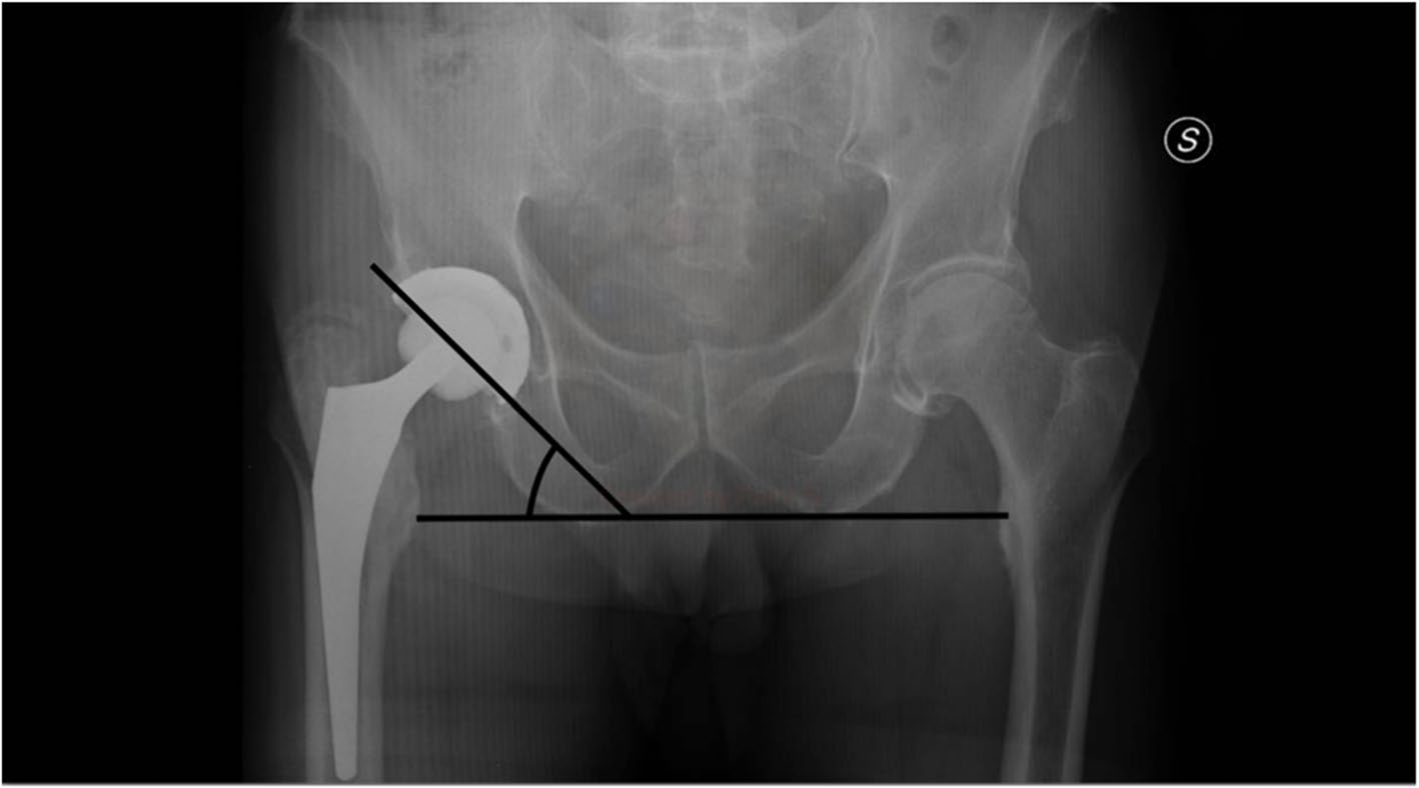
Acetabular cup inclination. Method of calculation of acetabular cup inclination on AP pelvic radiographs as the angle formed between a line drawn along the opening of the acetabular component and one joining the ischial tuberosities
